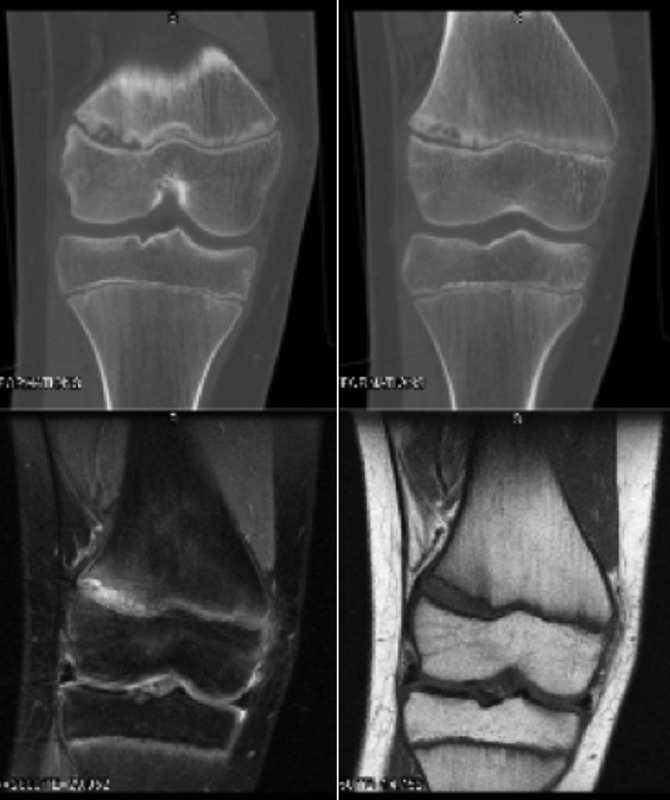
(A–D). CT and MRI sequences in an adolescent boy with atraumatic lateral distal femoral physeal arrest. CT demonstrates widening and fragmentation of the lateral physis (A, B). T2-weighted MRI sequences demonstrate increased signal and widening in the lateral distal femoral physis (C). T1-weighted images demonstrate physeal widening (D).
